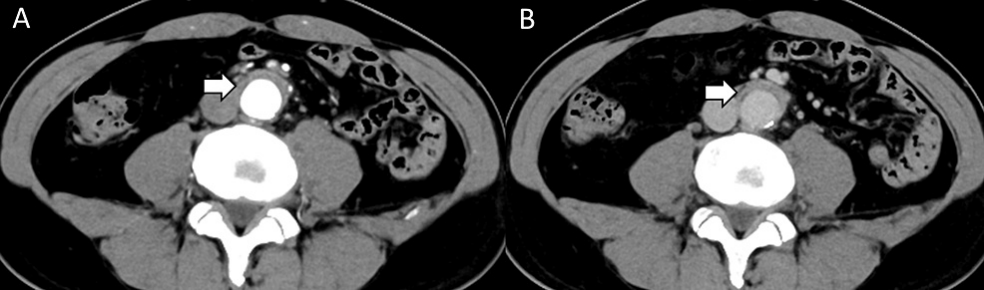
Periaortitis at the first visit. (A) Arterial and (B) equilibrium phase of contrast-enhanced computed tomography at the first visit: the abdominal aortic wall was thickened and periaortitis was suspected (arrow).
